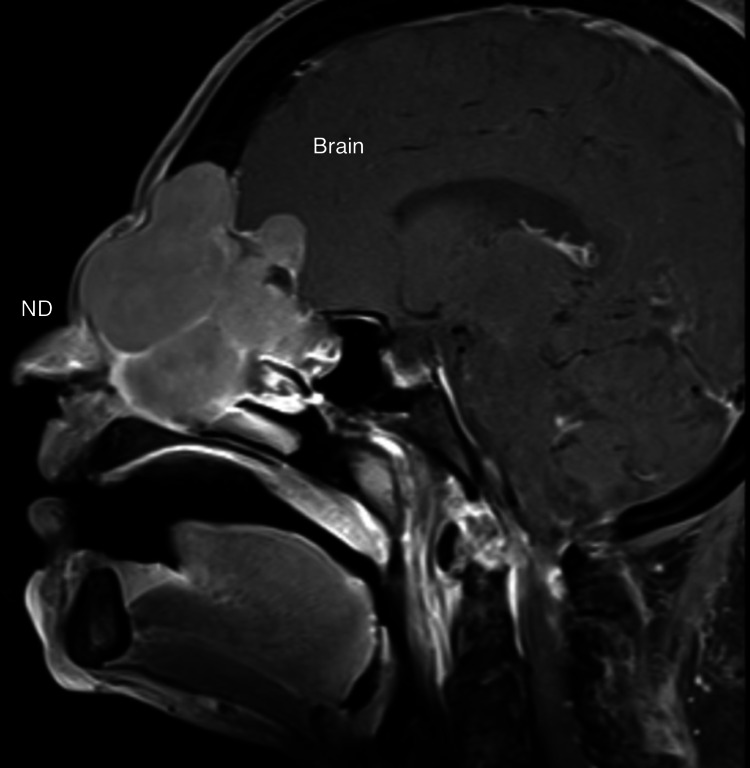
MRI on presentation, sagittal, T1 MRI with gadolinium, demonstrating mass effect on frontal lobe. Nasal dorsum (ND) is labeled. Enhancing mass does not erode though nasal dorsum or into oral cavity.
